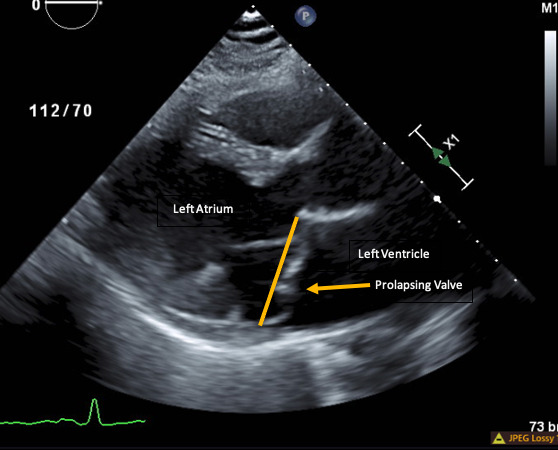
Echocardiographic parasternal long-axis view demonstrating mitral valve prolapse, characterized by systolic displacement of the mitral valve leaflets ≥2 mm beyond the plane of the mitral annulus.
